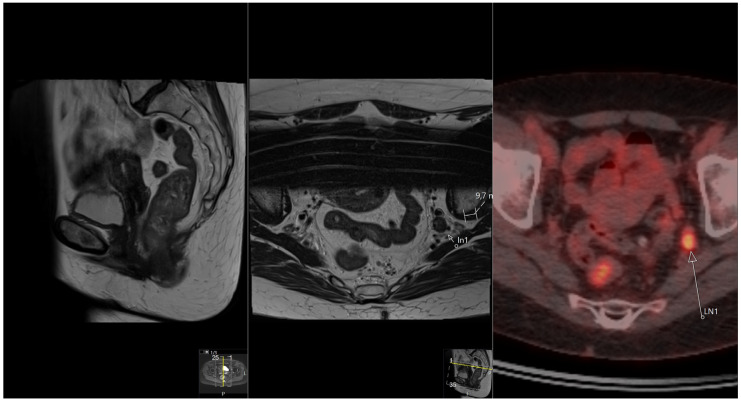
Nodular structure characterized on MRI as lymph node metastasis, located in the left lateral compartment. SUVmax = 5.7. Only the primary tumor is depicted on the sagittal image.
